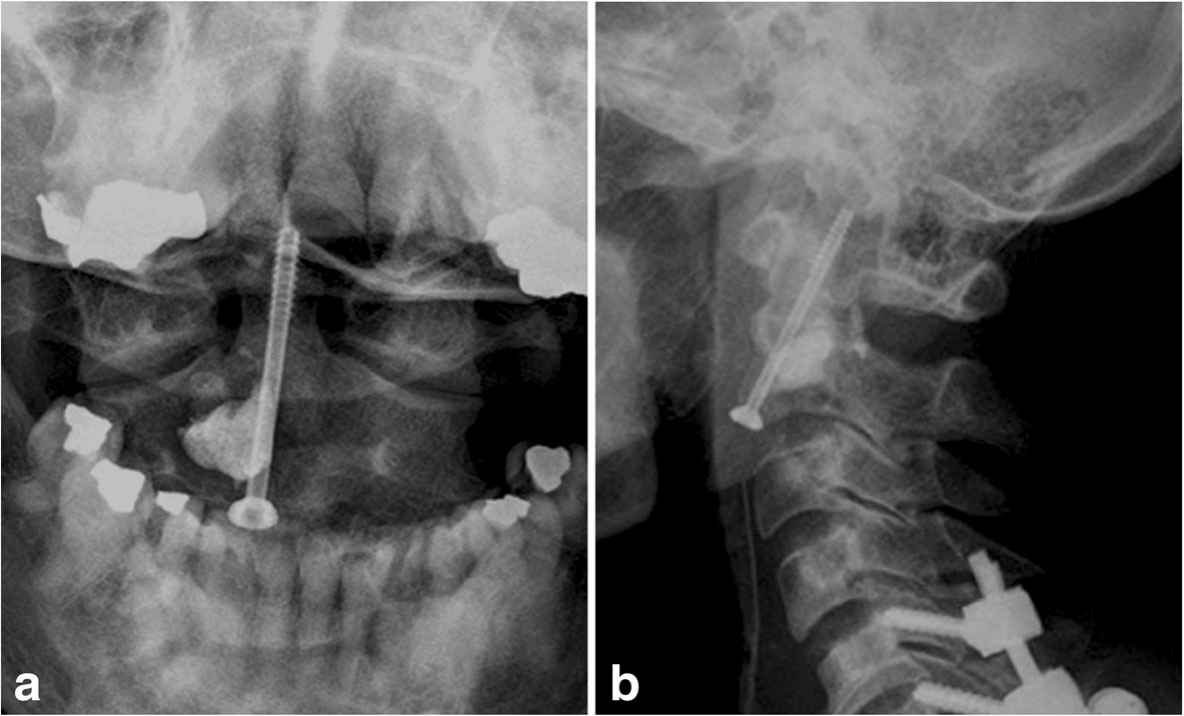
The postoperative x-ray shows cement in the base of C2 after balloon kyphoplasty and anterior odontoid screw (coronal view (a) and lateral view (b). Slight posterior cement leakage after kyphoplasty. Additional posterior instrumentation C5 to Th2 and laminectomy of C7 were performed for osteolytic lesion of C7 with compressed spinal cord (Case 1)
